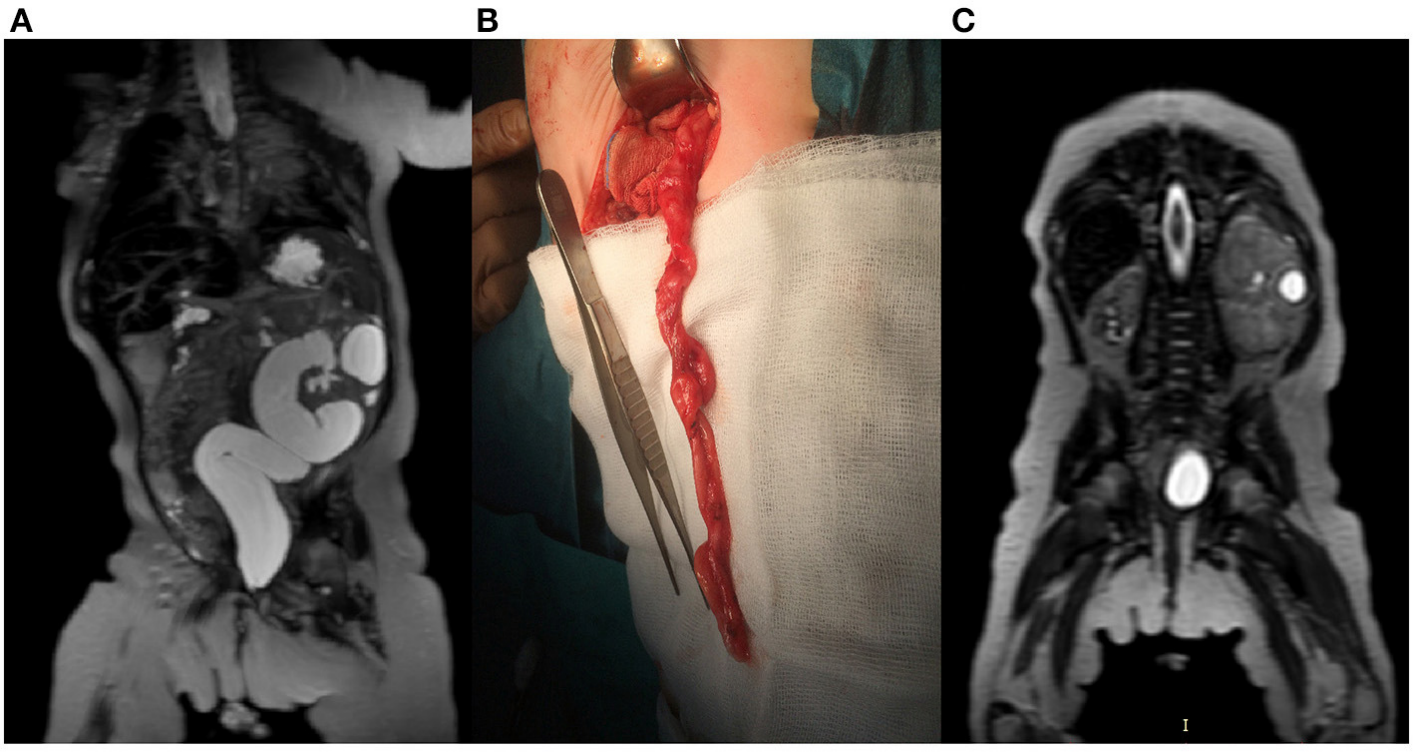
A 3-month-old female with left-sided megaureter. (A) T2-weighted image in the coronal plane shows left kidney with megaureter (B) Intraoperative picture of the partially excised megaureter. (C) T2-weighted image in coronal plane presents advanced dysplasia of the right kidney and localized dysplasia in the left, kidney.
